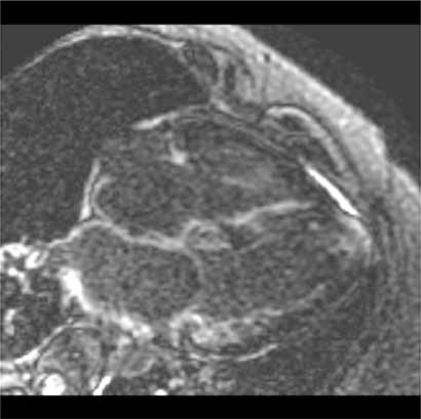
Cardiac MRI Showing Amyloidosis.
